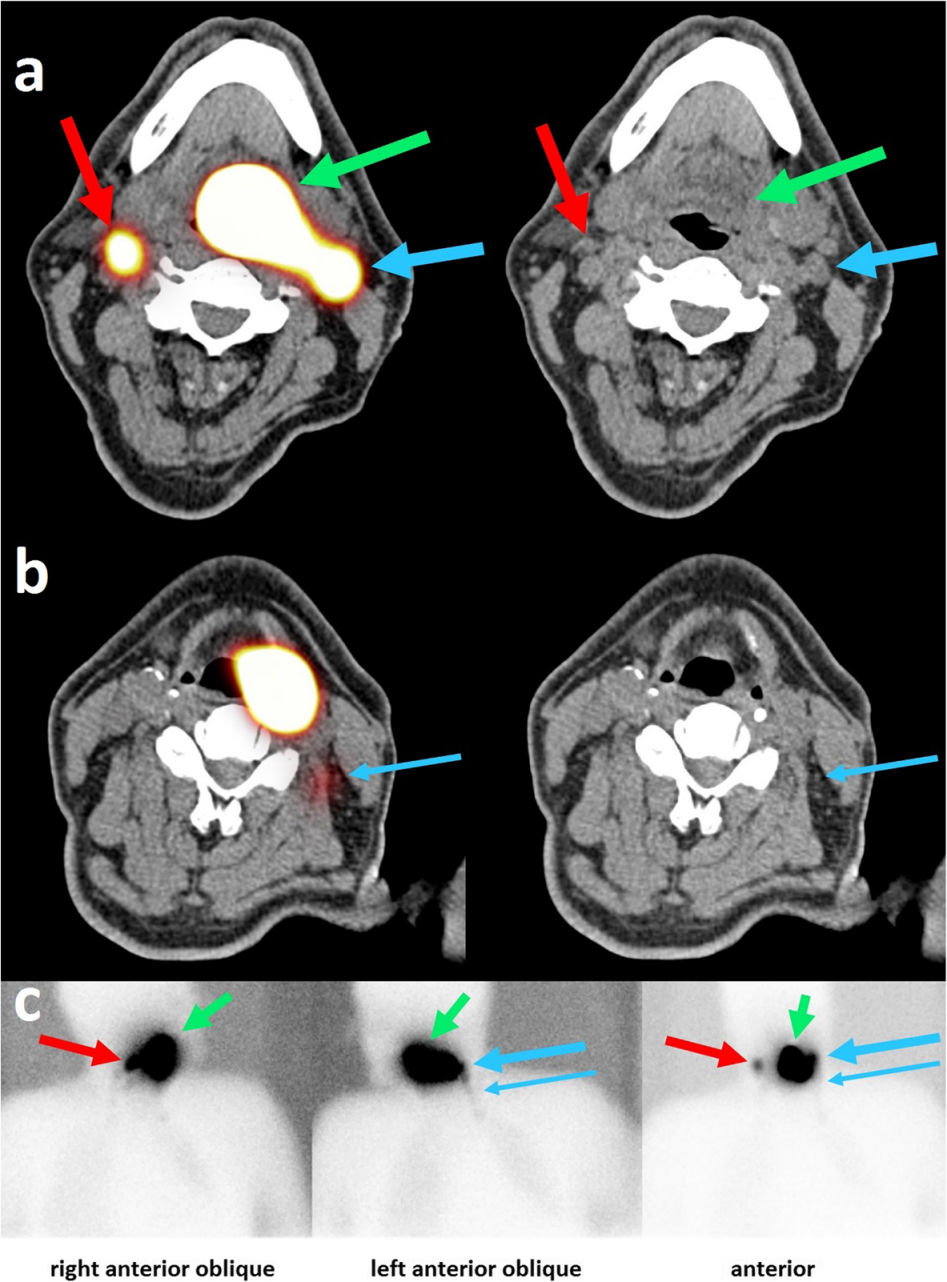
Example of SPECT/CT images. SPECT/CT images (a, b) and planar lymphoscintigraphy images (c) of a 64 year old patient with a T1 N1 base-of-tongue carcinoma. Fused SPECT/CT images are shown on the left panel, CT images are shown on the right panel. On the SPECT/CT images, ^99m^Tc-nanocolloid tracer accumulation is visible, indicating the primary tumor (green arrow), the first ipsilateral draining area in level 2 (large blue arrow), and the decreasing tracer activity down the ipsilateral nodal chain into level 3 (small blue arrow). Furthermore, a contralateral draining area is visible in level 2 (red arrow). In the SUSPECT-2 study, this patient would be a candidate for a contralateral sentinel node procedure on the same day as the lymph drainage mapping. On the CT images, the arrows point to the lymph nodes that are thought to be the anatomical substrates that correlated with tracer accumulation
